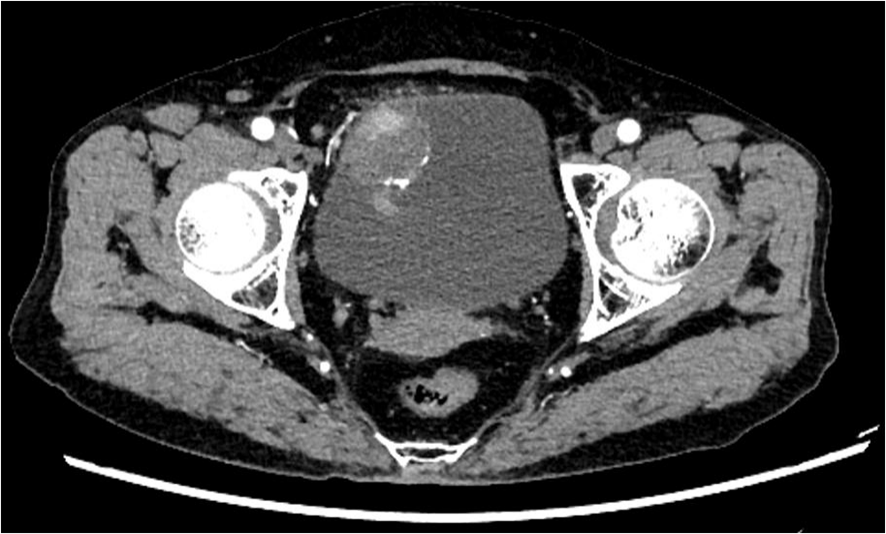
CT Abdominal Scan: Present within the bladder is a quasi-circular soft tissue mass of low density. Observed in the enhanced scan is inconsistent enhancement, and the demarcation with the anterior wall of the bladder remains obscure.
